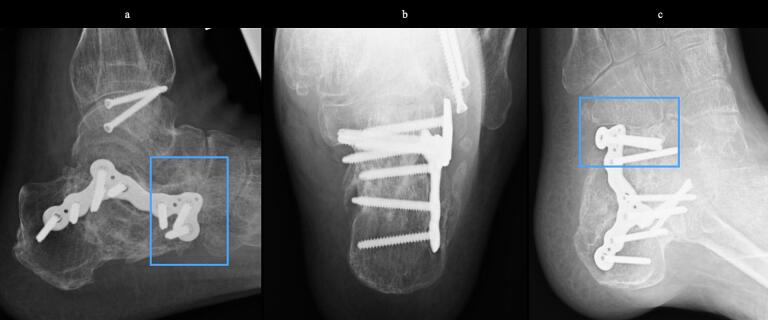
X-ray 7 months after surgery. Bone union without correction loss. a: lateral view of the calcaneus. b: axial view of the calcaneus. c: Oblique view of the foot.
